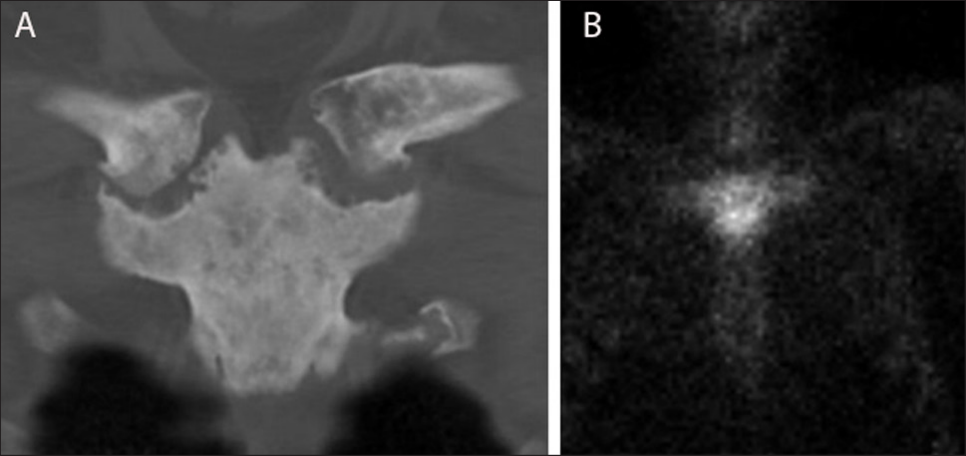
Typical bullhead sign in SAPHO of the sternoclavicular joint. CT (coronal reformatted image) (a) shows sclerosis of the manubrium sterni and medial clavicles and erosions of the sternoclavicular joints. Note a typical bullhead sign on scintigraphy (b).
